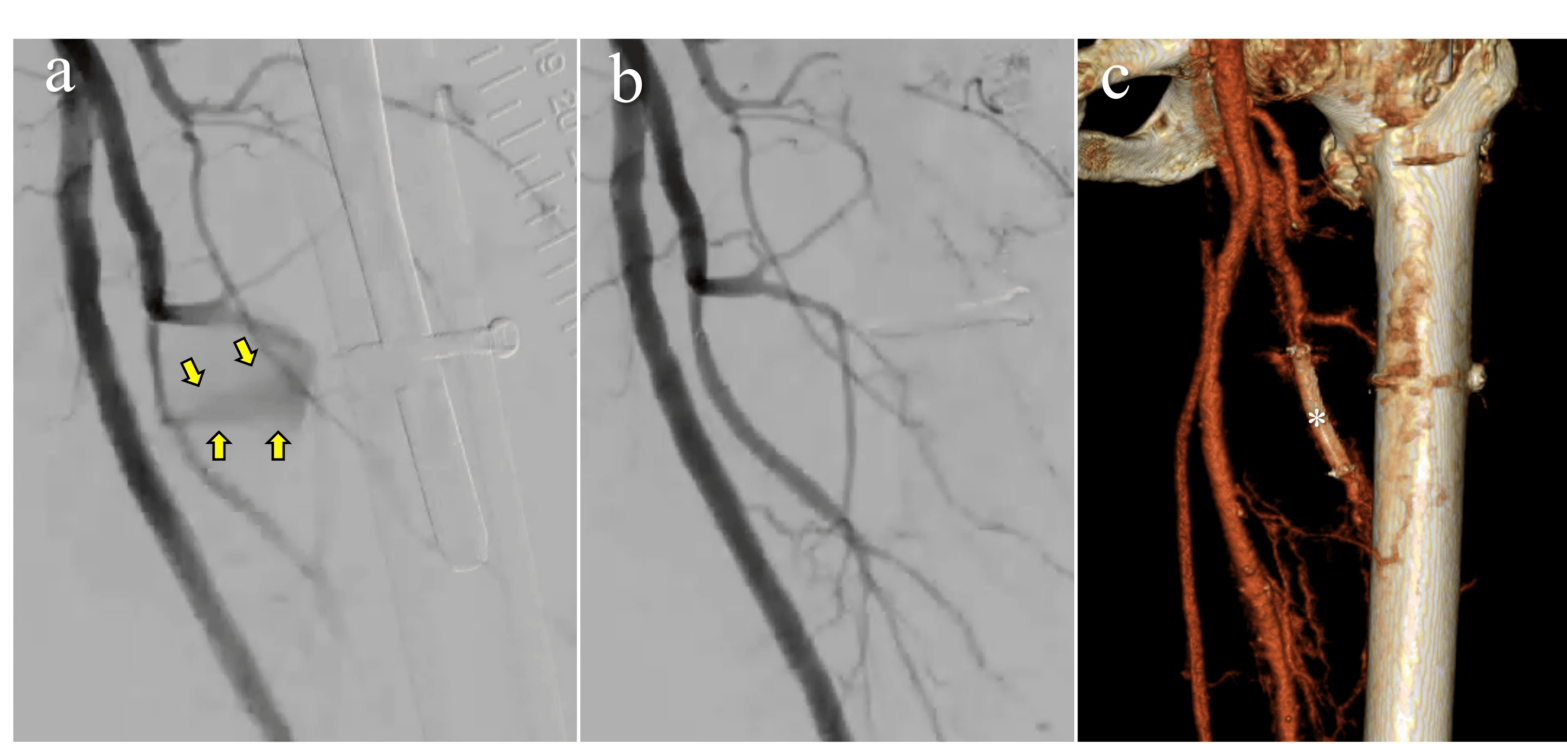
Angiographic findings before and after stent grafting (a) Digital subtraction angiography before treatment showing contrast extravasation from the deep femoral artery (yellow arrows). (b) Posttreatment angiography confirming successful hemostasis and restoration of blood flow. (c) Three-dimensional computed tomography angiography demonstrating the deployed stent graft (asterisk) in the deep femoral artery, with no visualization of the pseudoaneurysm
